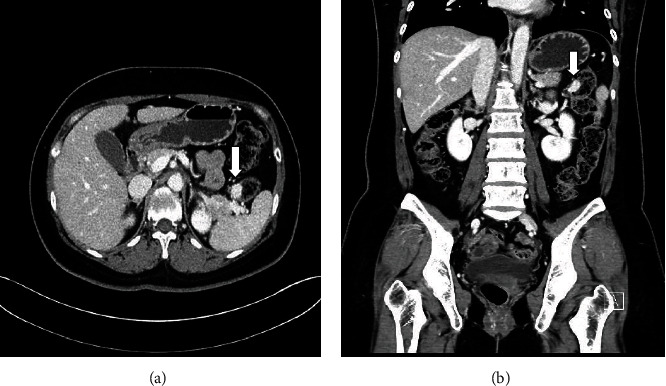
Radiologic findings. Contrast-enhanced CT image shows a 1.6 cm hypervascular tumor (arrow) in the splenic flexure: (a) transverse view and (b) coronal view.
